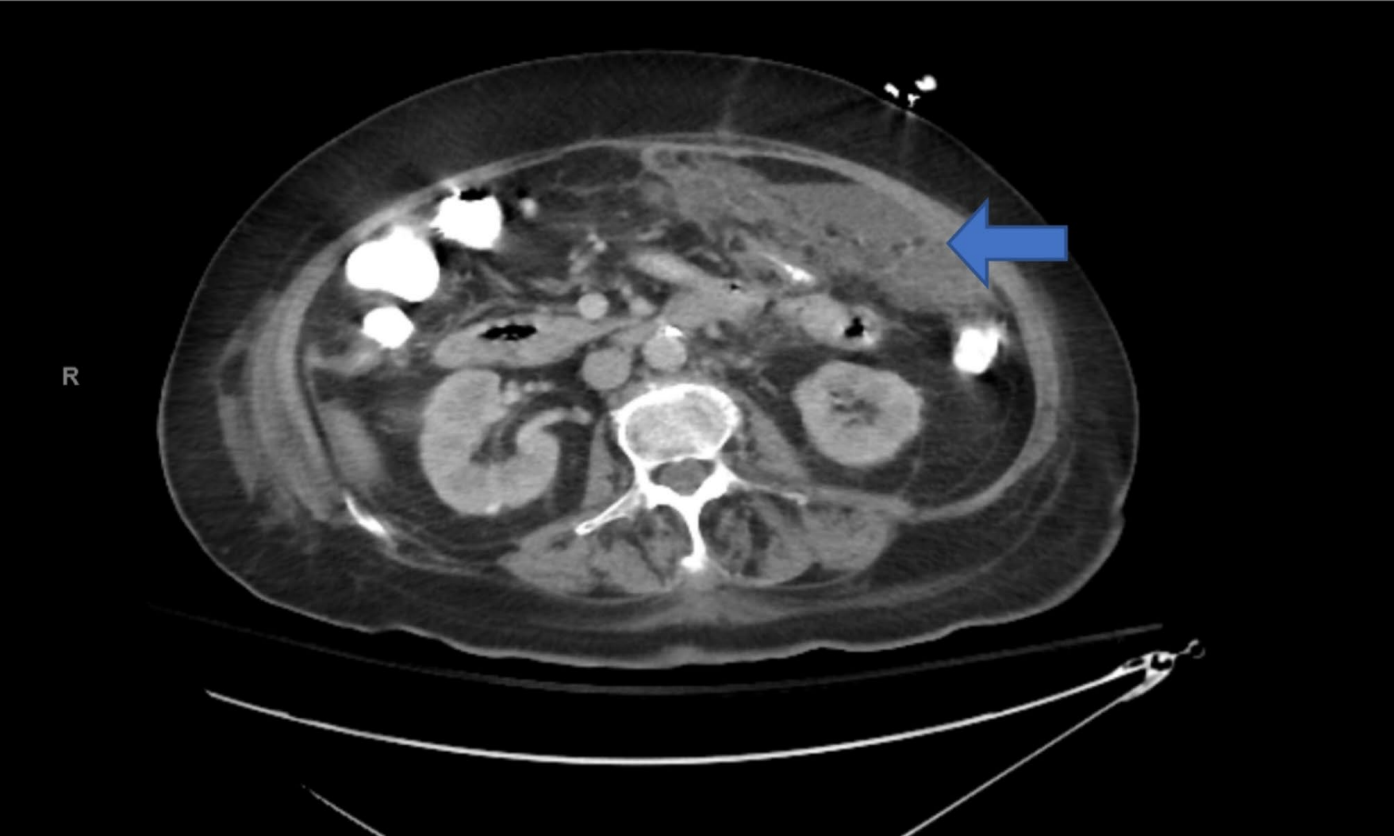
Axial CT section in a 71-year-old man with PTB revealing omental inflammation of the left peritoneum (indicated by the arrow)
